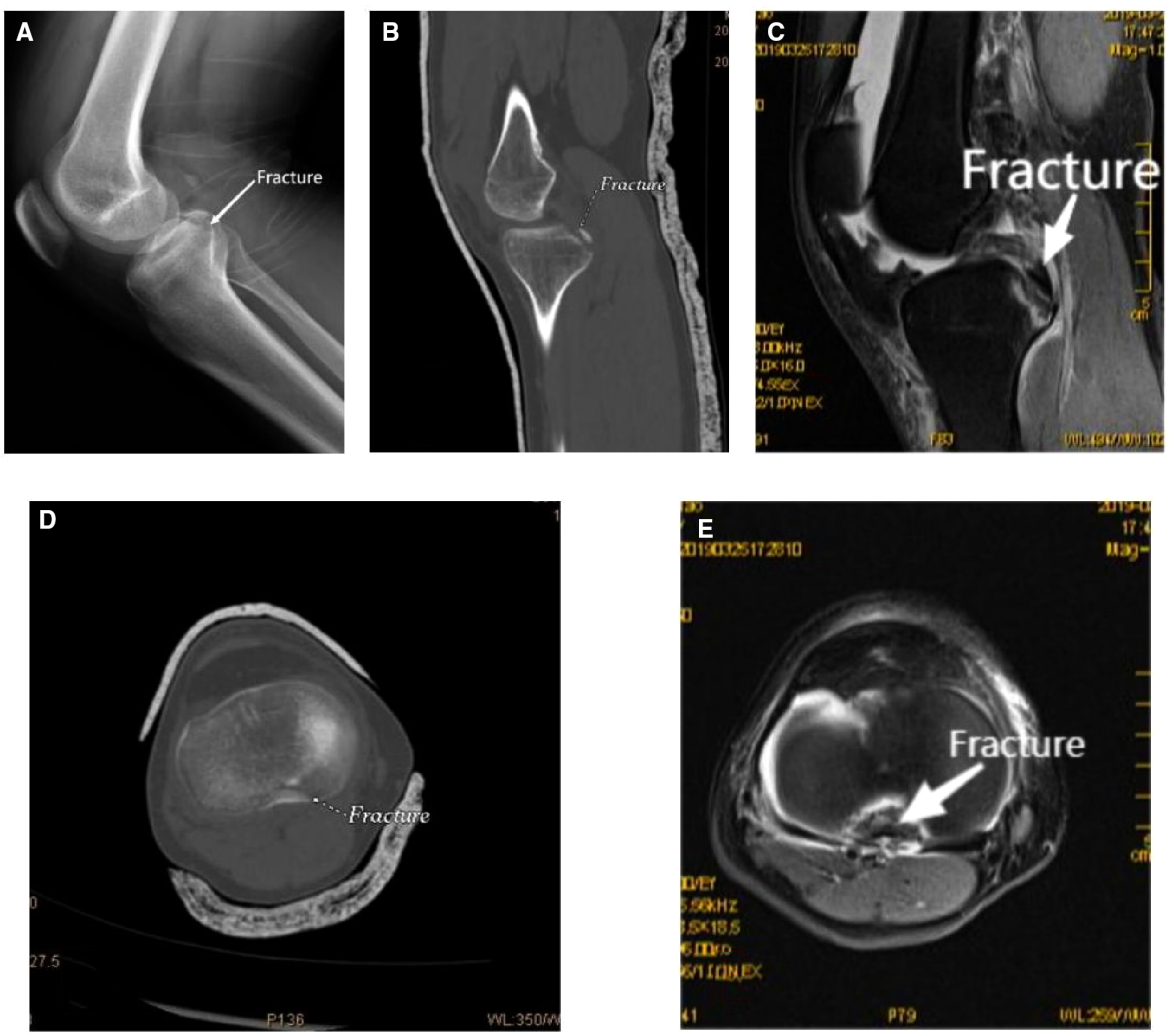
Preoperative x-ray, CT, and MRI of the patient [(B,C) sagittal view; (D,E) transverse view]. CT, computed tomography; MRI, magnetic resonance imaging.
